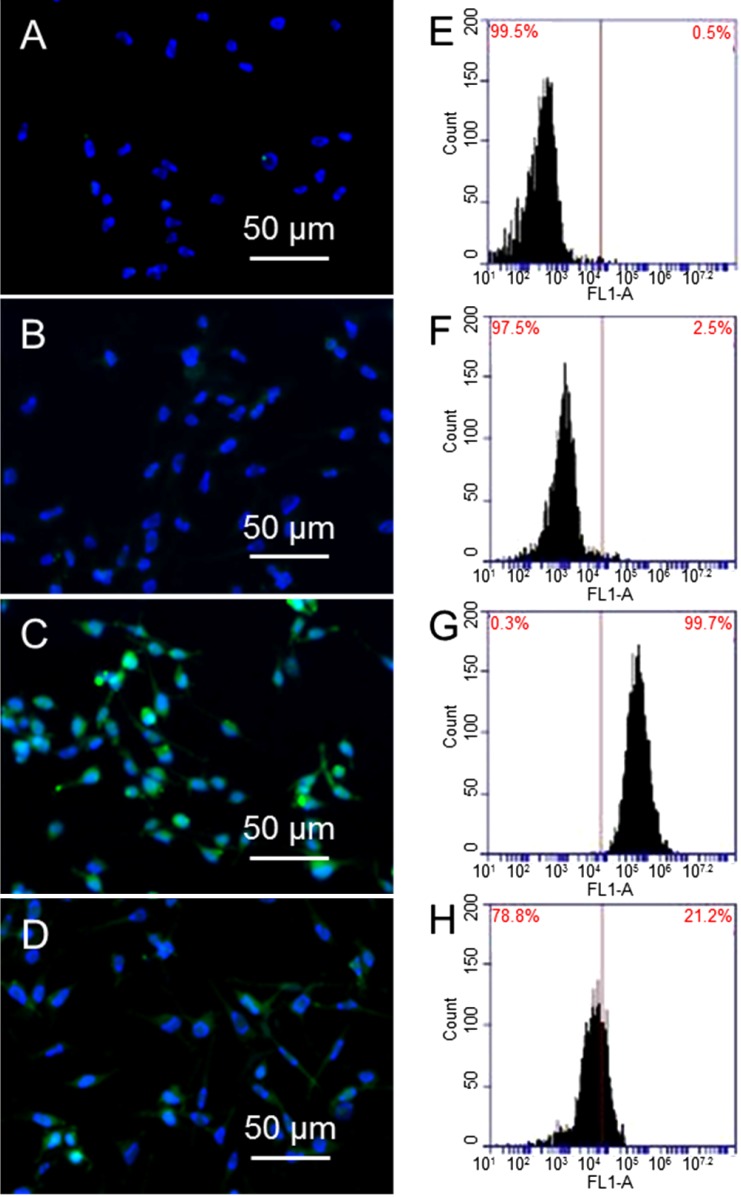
Oxidative stress induced by GO and X-ray. Fluorescent images of cells (A); cells treated with 10 µg/mL GO (B); cells exposed to 1.25 Gy X-ray (C); and cells treated with 10 µg/mL GO and then exposed to 1.25 Gy X-ray (D); Flow cytometry results of cells after different treatment: cells (E); cells treated with GO (F); cells exposed to 1.25 Gy X-ray (G); and cells treated with GO and exposed to X-ray (H).
